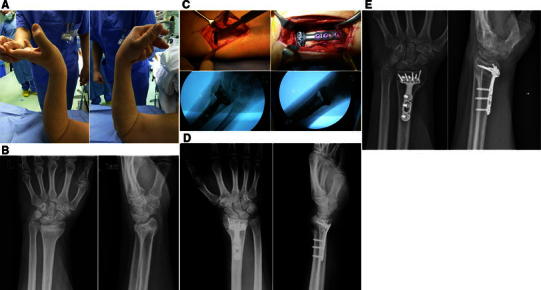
Case 1. a Preoperative range of movement evaluation. b Preoperative X-rays showing the dorsal inclination of articular surface of the distal radius.  c Intraoperative view showing the osteotomy of the radius, the synthesis and the final correction under fluoroscopy. d X-rays 30 days after surgery showing the dorsal gap with early signs of refilling. e Good healing of the osteotomy with complete refilling of the dorsal gap at 3 months after surgery
